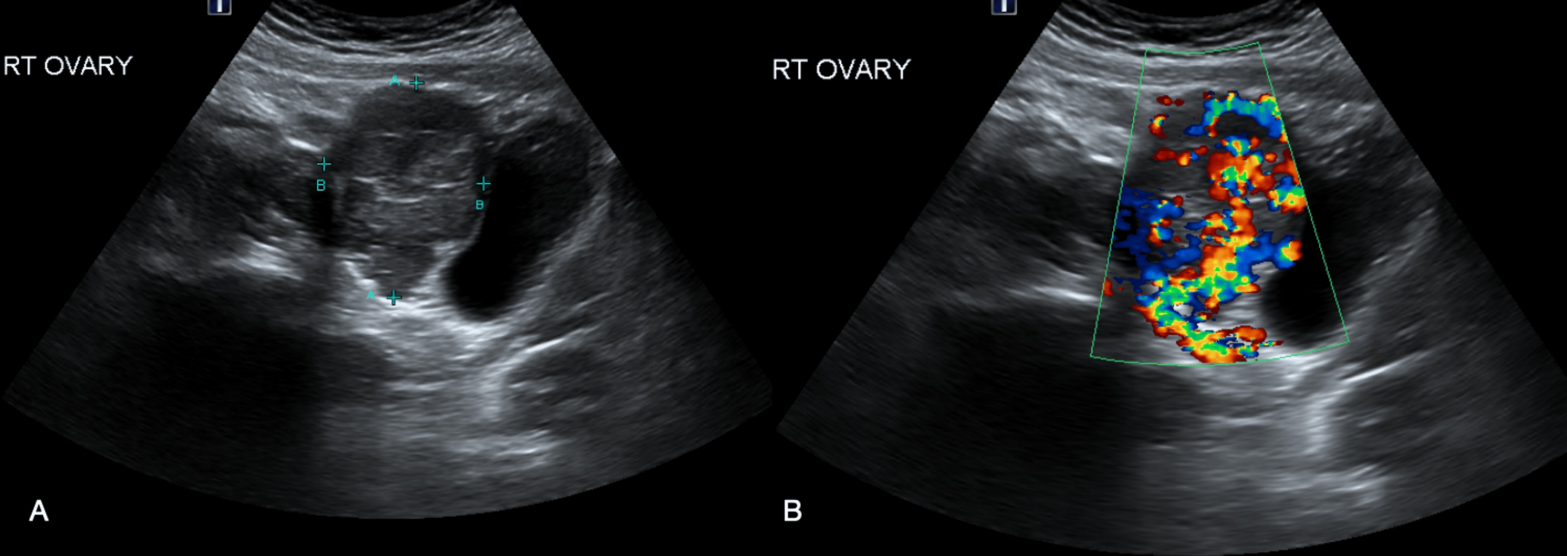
Ultrasound (pelvis) shows (A) well-defined, solid hypoechoic mass in the right adnexa and (B) hypervascularity on color doppler imaging.
